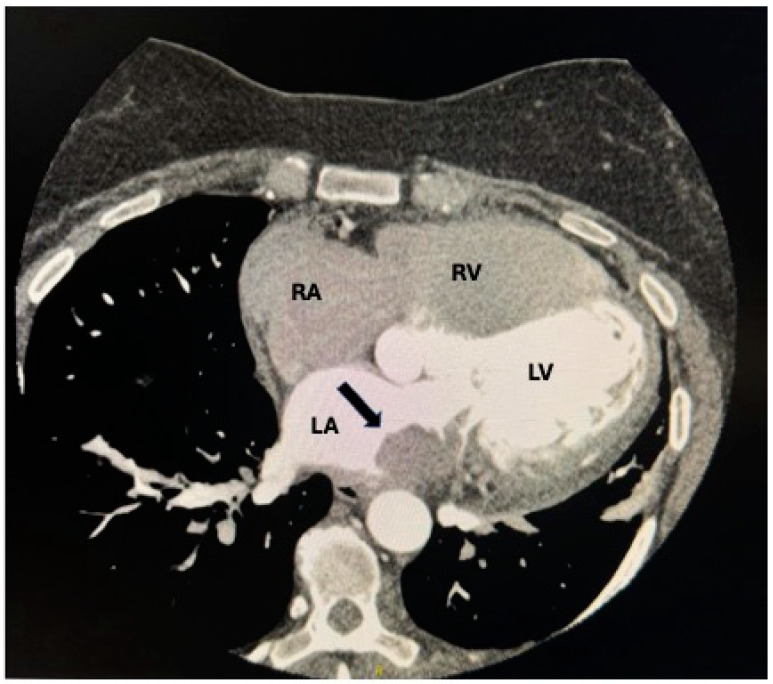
A contrast-enhanced CT image showing an endoluminal mass in the left atrium, likely metastatic from renal carcinoma. The black arrow indicates pathological tissue within the left atrium during the arterial phase following iodinated contrast infusion. RA: right atrium; LA: left atrium; LV: left ventricle; RV: right ventricle.
